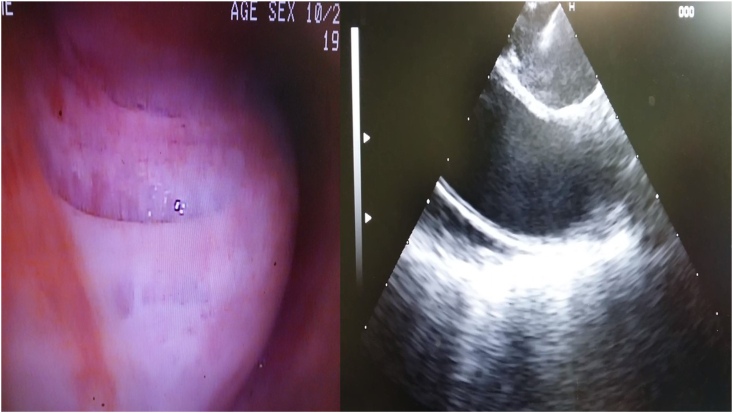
From left to right: Left; is the endoscopic view of the left stem bronchus, right; is the endosonography performed from the EBUS-TBNAB 19G needle puncture.
